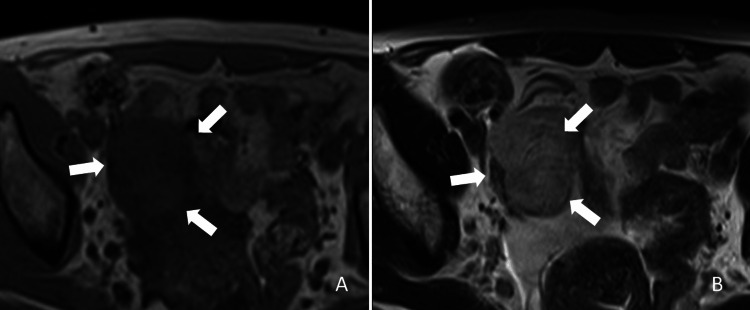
Magnetic resonance images, (A) T1-weighted image, and (B) T2-weighted image. The right adnexal mass shows an iso-signal intensity on the T1-weighted image (A, arrows) and heterogeneously high signal intensity (B, arrows) on the T2-weighted image in comparison to the skeletal muscle.
